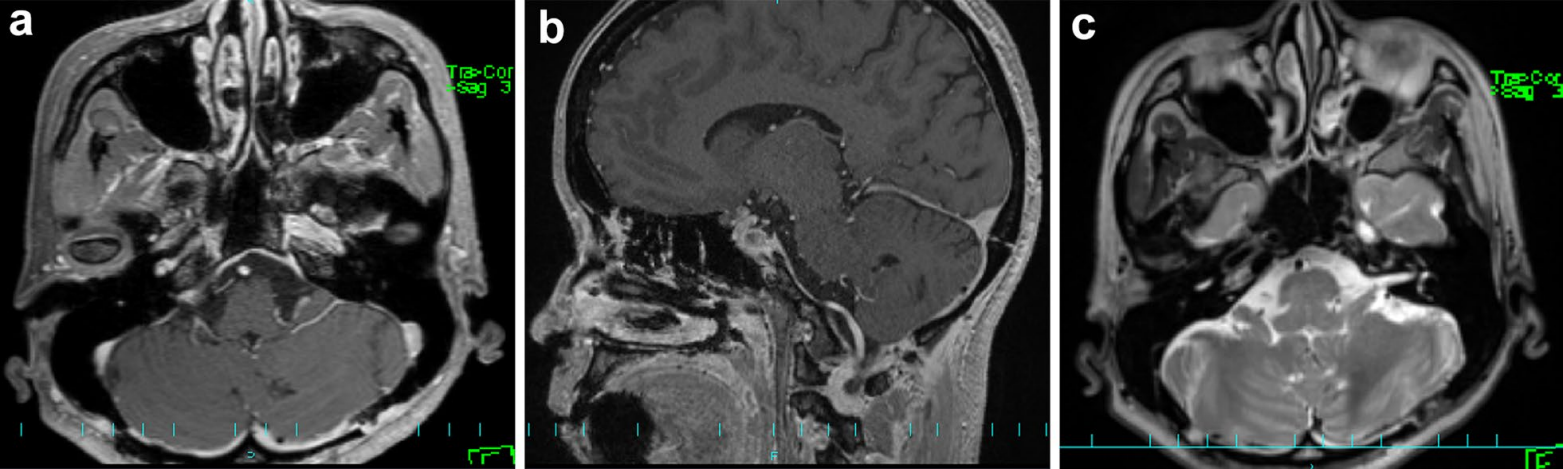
Postoperative magnetic resonance imaging. Postoperative axial (a) and sagittal (b) views of gadolinium-enhanced T1-weighted and c T2-weighted MRI, showing total resection of the tumor and no ischemic and contusional complications
